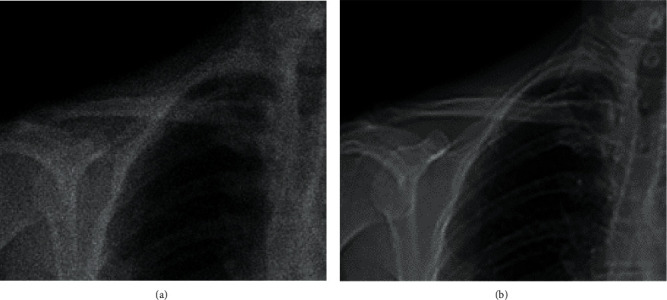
Comparison of the effect of scapula CT before and after artificial intelligence enhancement. (a) Unenhanced CT image of the scapula. (b) Enhanced CT image of the scapula.
